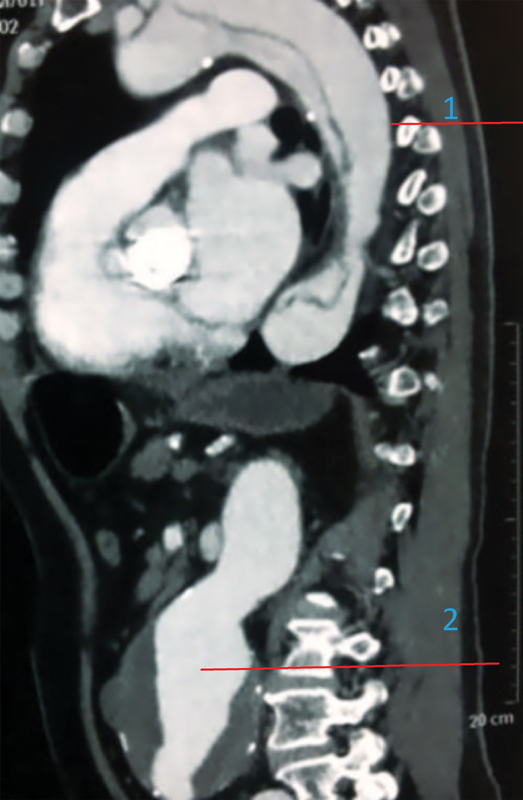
Sagittal computed tomography (CT) scan that documents aortic dissection (Type A) and aortic aneurysm. 1, aortic dissection with the true and the false channel; 2, aortic aneurysm.
